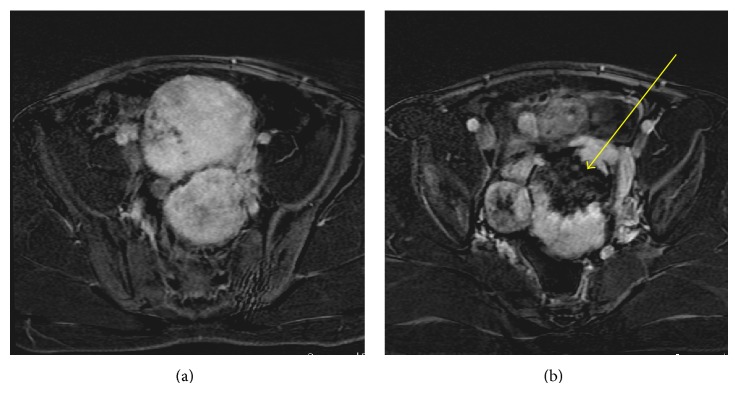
MRI imaging. (a), (b) Axial postgadolinium images demonstrate areas of significant enhancement within the pelvic masses with a central area of nonenhancement (*yellow arrow*) corresponding to the area of T1 hypointensity, which represents the fibrous portion of the tumor.
